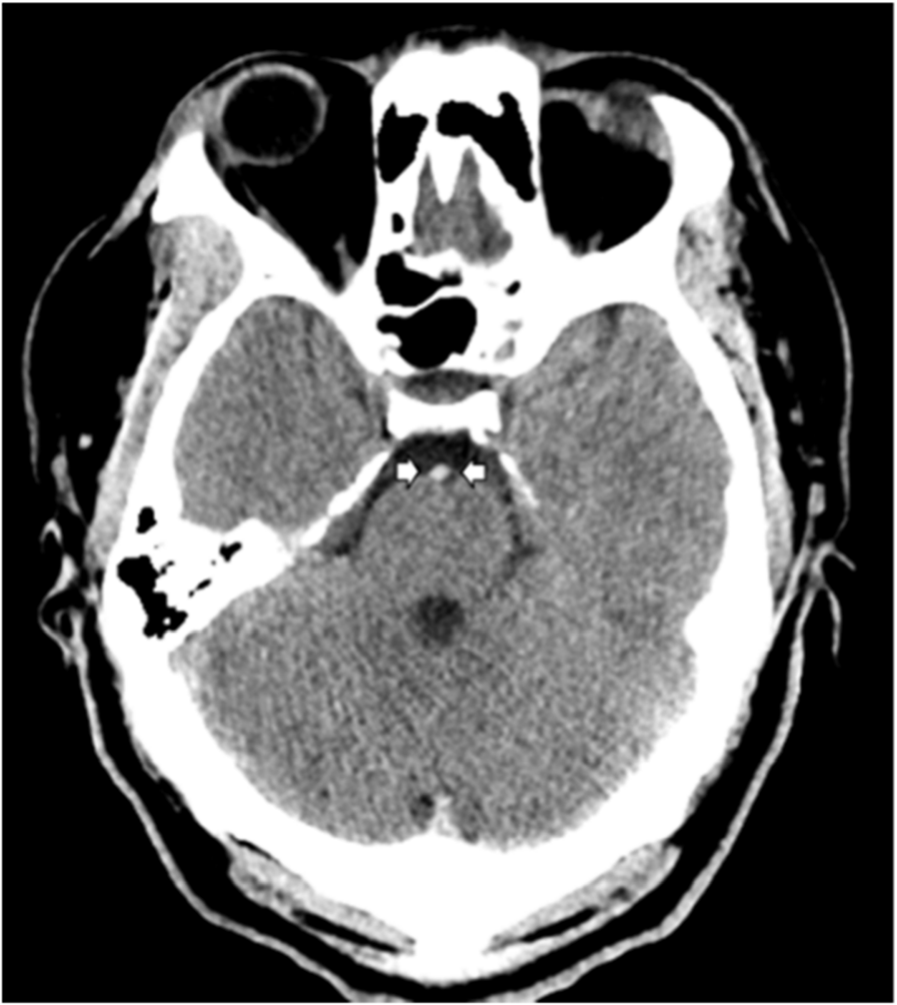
Non-contrast CT scan with hyperdense basilar artery sign (arrows). No hypodensities were identified in the brainstem, cerebellum, or cerebral hemispheres
